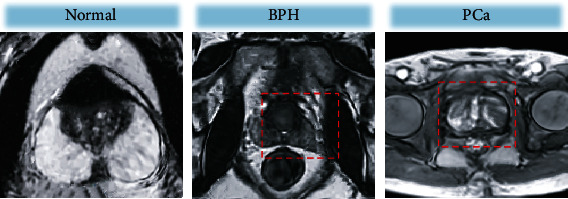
DWI scanning images. The red circles showed the lesions.
